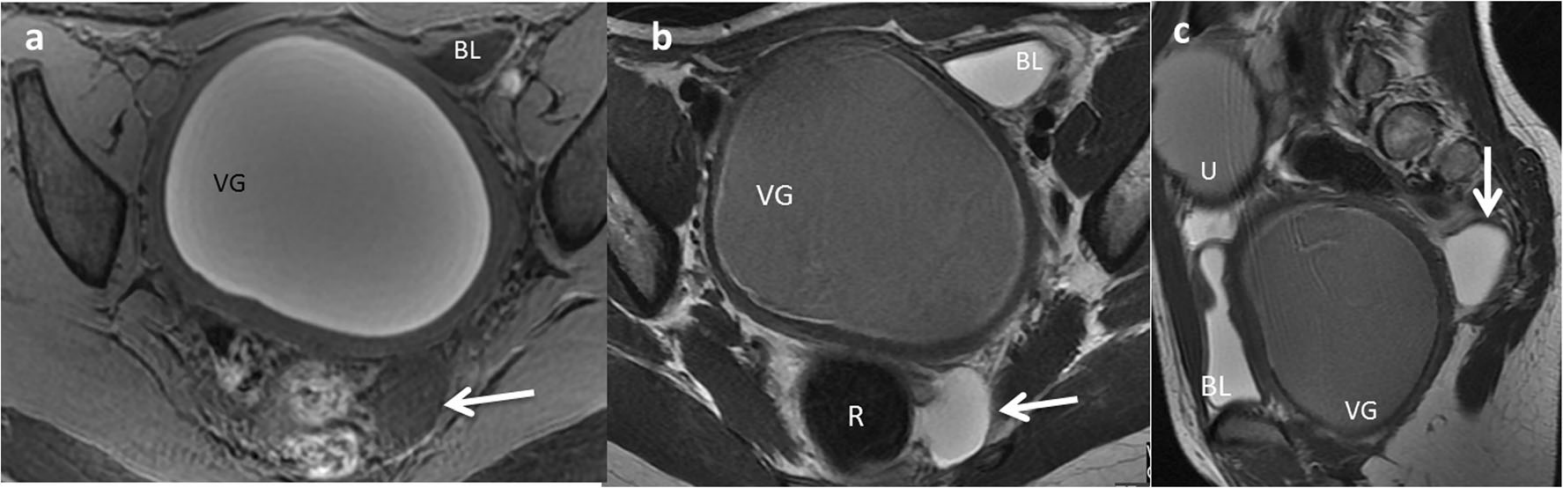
A 12-year-old girl with a gynaecological malformation and haematometrocolpos. a A hypointense lesion is seen (*white arrow*) next to the rectum in axial T1 weighted pelvic MR. b On T2-weighted pelvic MR, the cyst next to the left wall of the rectum is seen. c Sagittal plane of the T2 MRI showing the posterior location of the cyst (*arrow*). *VG* vagina, *BL* bladder, *U* uterus. Imaging findings of a rectal duplication cyst. Note the haemorrhagic content of the uterus and vagina because of haematometrocolpos
